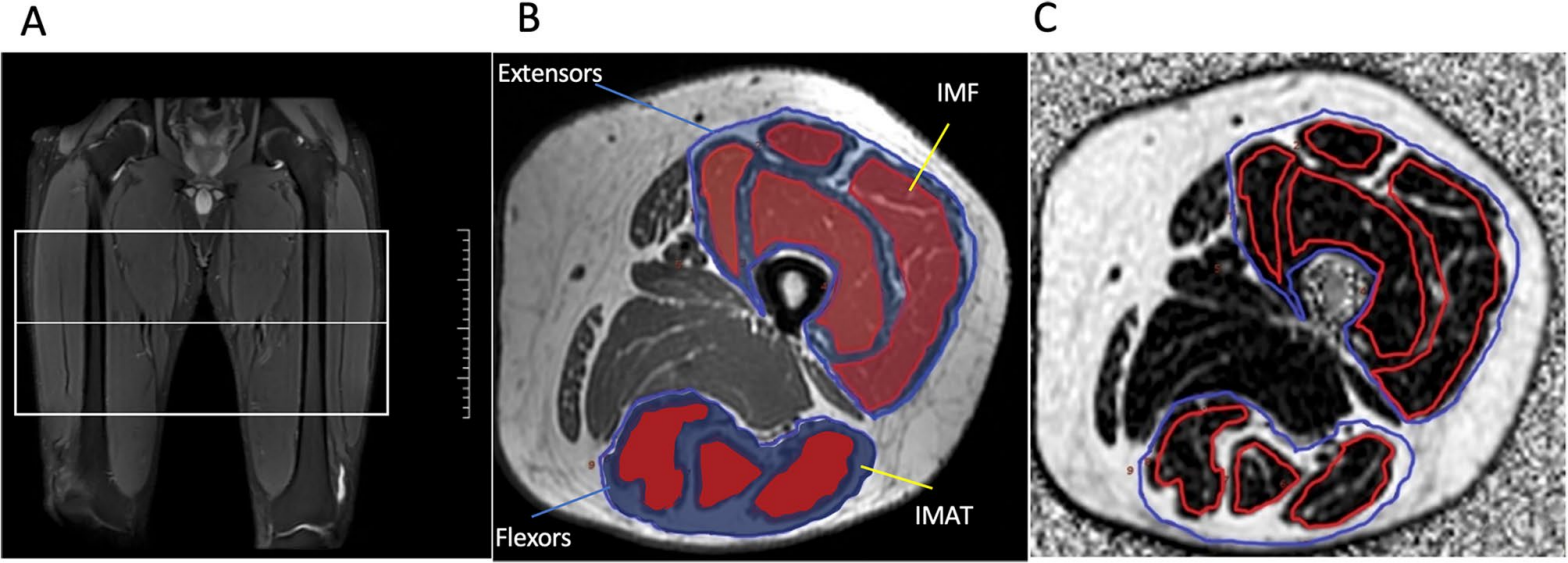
Magnetic resonance image scanning and muscles MRI ROI delineation. (A) MRI scanning range of the thigh (white box). (B) Axial T1WI showing segmentation of thigh muscle and fat. IMF, intramuscular fat; IMAT, intermuscular adipose tissue. (C) PDFF maps of left thigh muscle.
